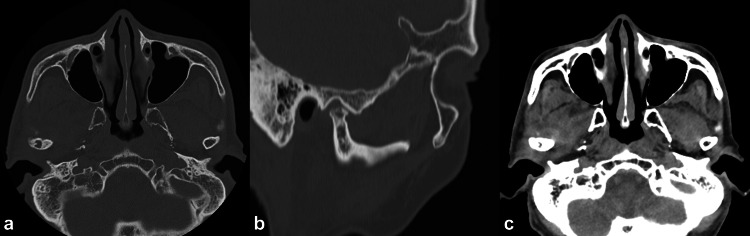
Computed tomography images. (a) Axial image of the bone showing coarse resorption of the right mandibular condyle with surrounding sclerosis. (b) Sagittal image of the bone showing coarse resorption of the right mandibular condyle with surrounding sclerosis and osteophyte formation. The articular fossa and eminence were relatively preserved. (c) Axial image of the soft tissue showing thickened soft tissue surrounding the right mandibular condyle.
